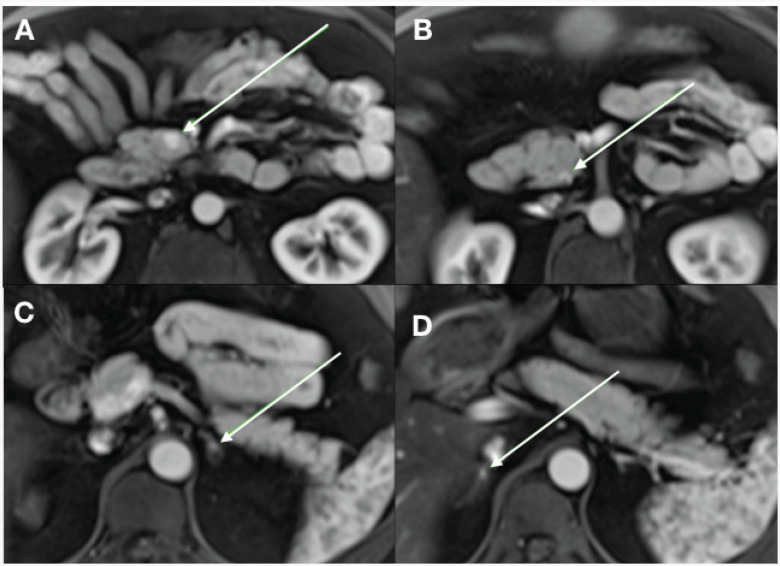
Abdominal magnetic resonance imaging showing two pancreatic neuroendocrine tumors (A and B) in the presented case and the pheochromocytoma lesions on the left (C) and (D) right sides.
